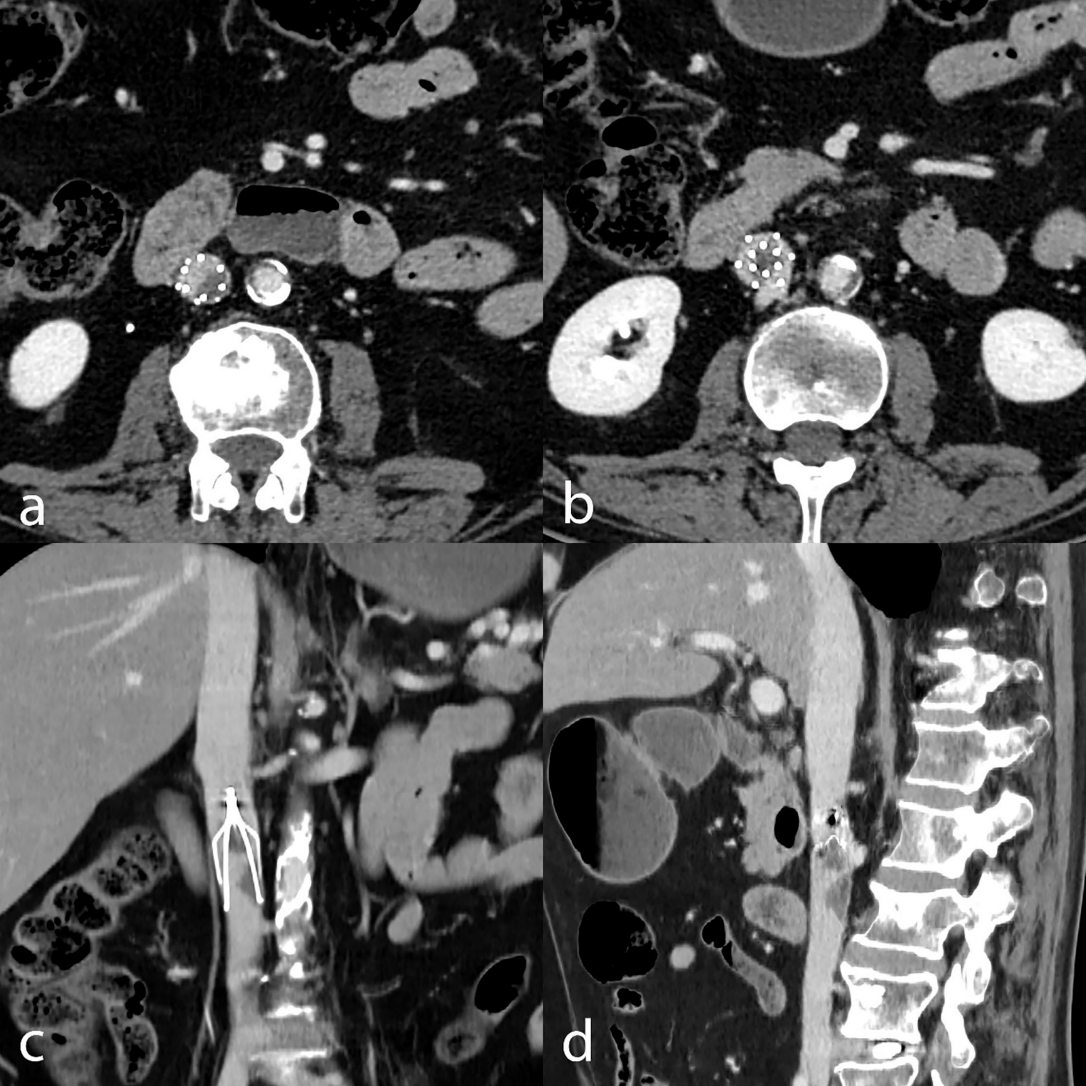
Technical irretrievability due to thrombus inside the filter. CT venography with axial (a,b), coronally reformatted (c), and sagittally reformatted minimum-intensity projection (d) images show thrombotic material stuck in the filter, precluding retrieval.
